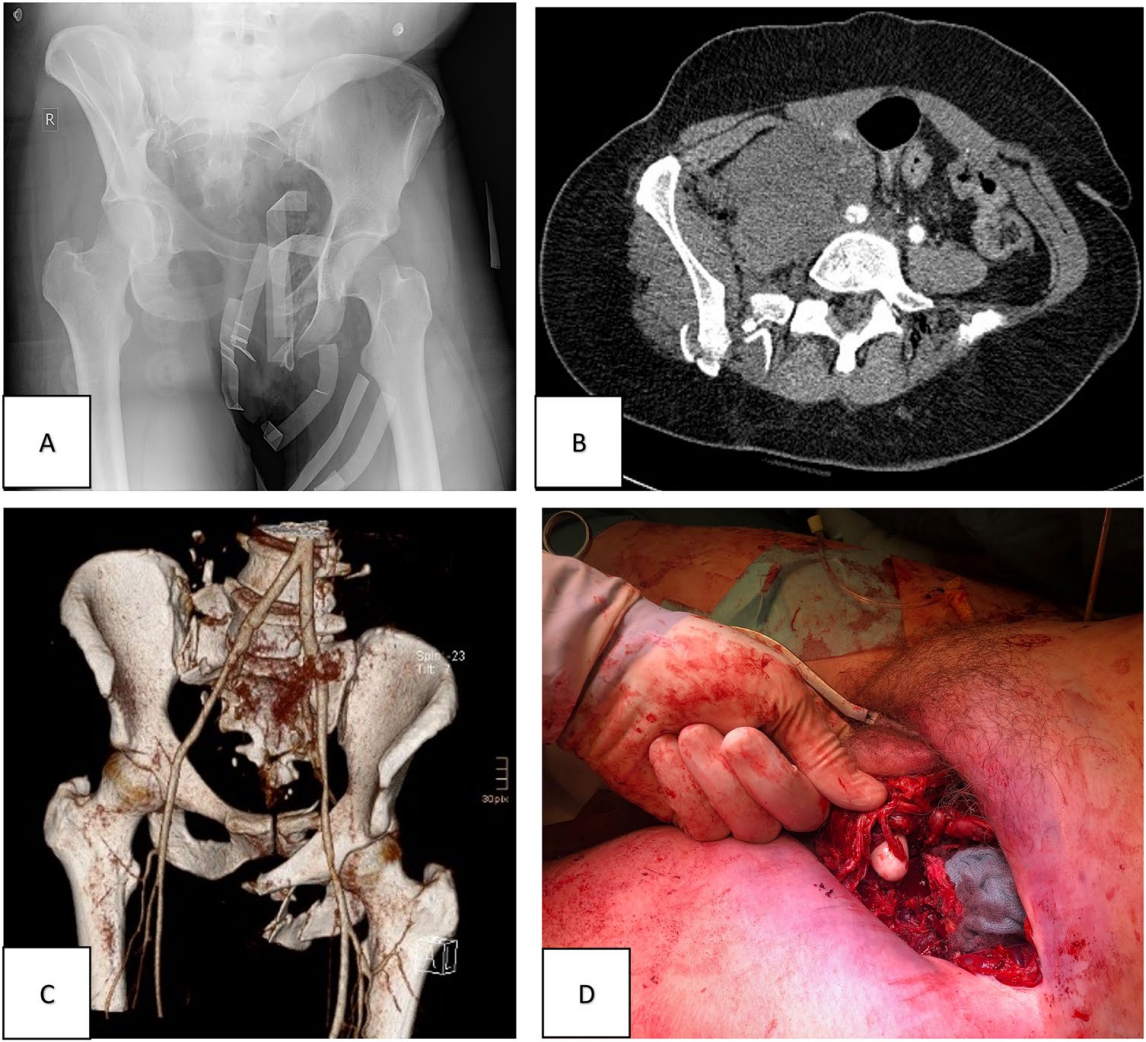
Patient 1 initial diagnostics and intraoperative findings. A Initial X-ray of the pelvis obtained in the emergency department under ongoing cardio-pulmonary resuscitation. B MS-CT scan with contrast showing a traumatic dissection of the right common iliac artery. C MS-3D-CT scan with contrast of the obtained pelvic injury (61-C3.2 c, h, j acc. to AO; right sacral fracture, left partial iliosacral disruption; partial traumatic hemipelvectomy), dissection of the common iliac artery and bleeding from the internal iliac artery on the right side. D Intraoperative findings revealing a tear of the femoral nerve
